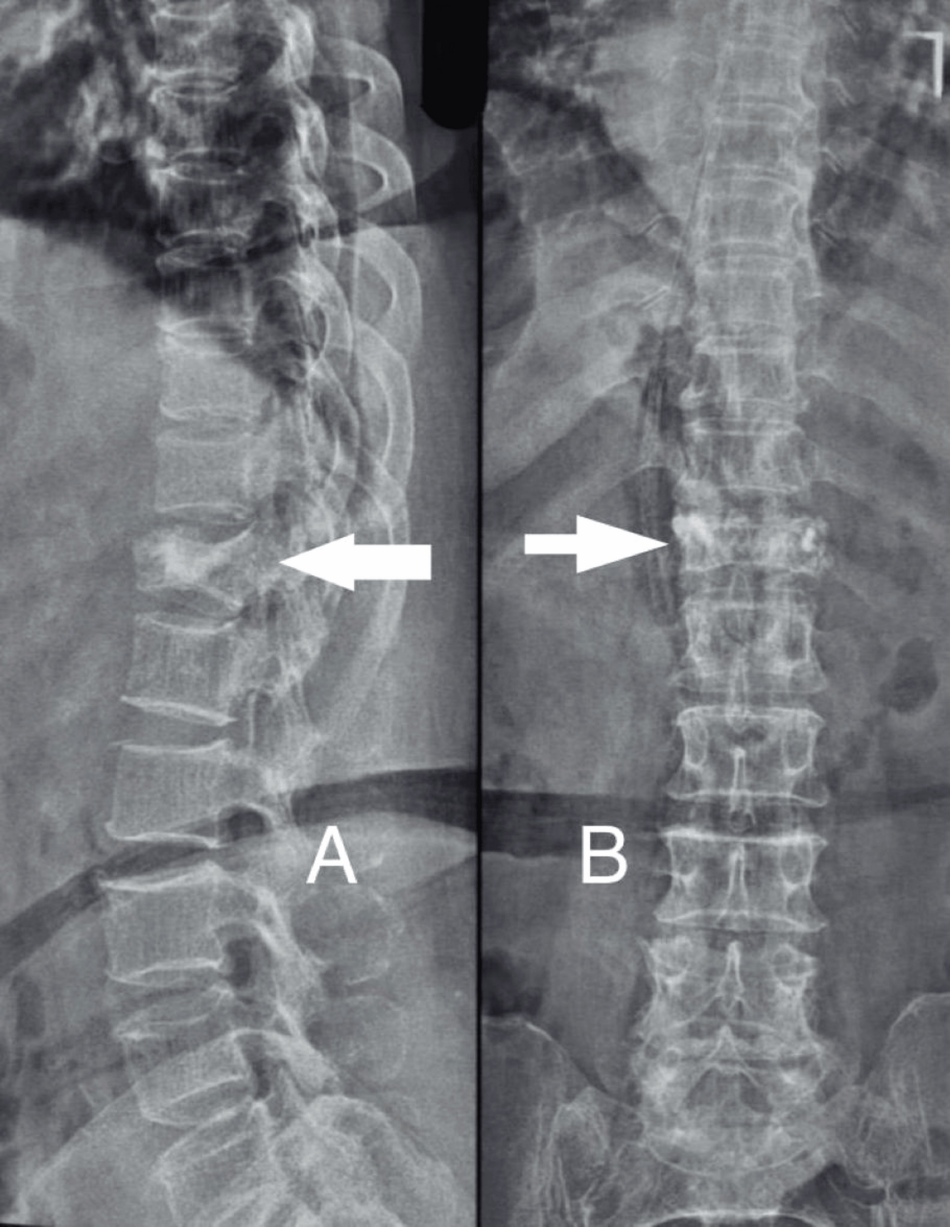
A) postoperative X-ray of same patient showing Lumbosacral spine lateral view with bone cement in D12 vertebrae; B) postoperative X-ray of same patient showing lumbosacral spine anteroposterior view with bone cement in D12 vertebrae
